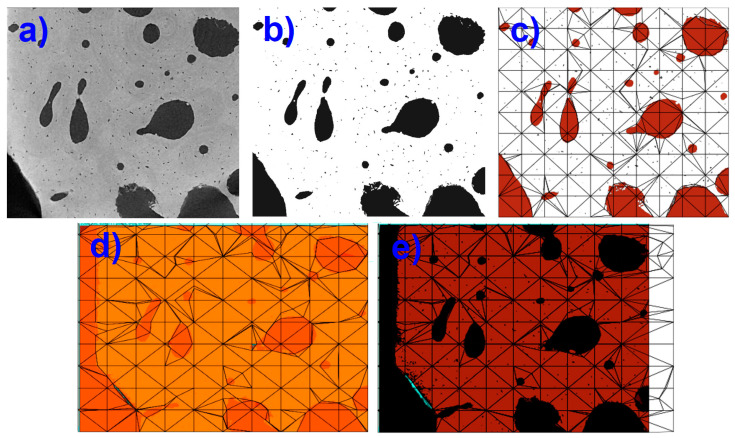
Displacement field generated in the cortical bone structure. (a) CT scan imagen. (b) Digitization of images for phase separation. (c) Mesh displacement. (d) Displacement: −8.04 mm. (e) Displacement: −18.09 mm.
